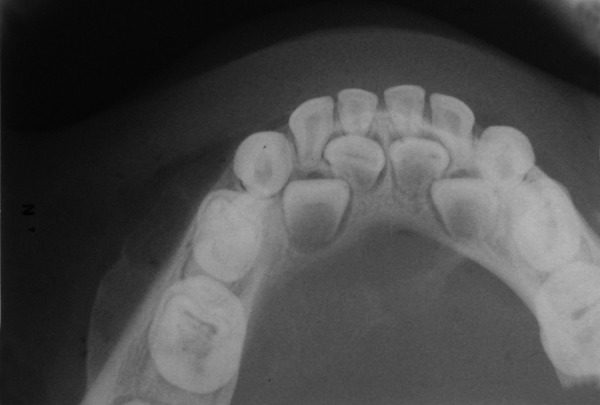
Occlusal radiograph showing well-defined radiolucency crossing midline with thinning of the buccal and lingual cortical plates
